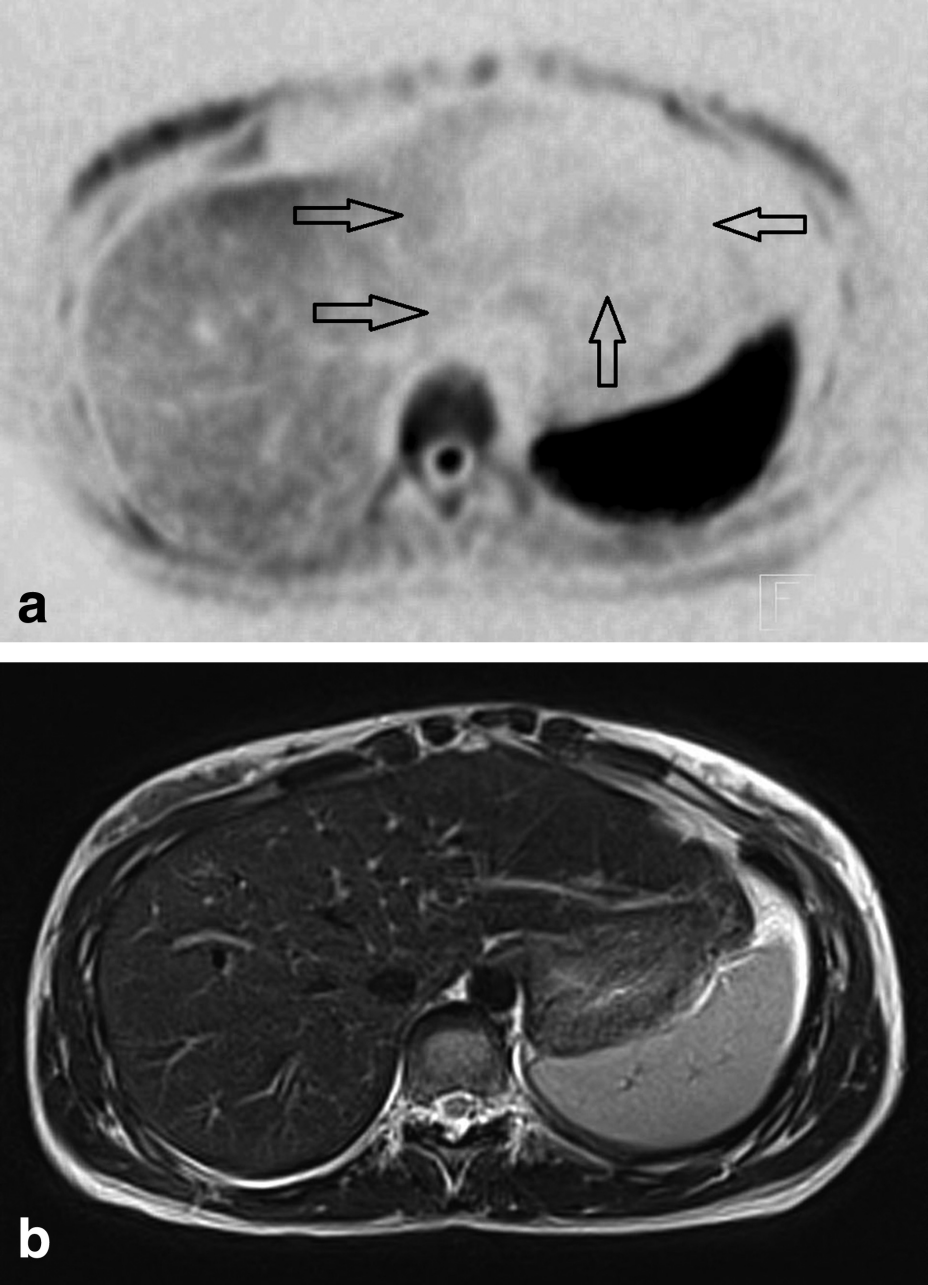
Axial b-500 image (a) demonstrates loss of signal within the left aspect of the liver from cardiac pulsation. The anatomy is not obscured on the axial T2 (b) image. This loss of signal is less problematic at low b-values and can be practically minimized by increasing the number of averages for DWI, or cardiac gating.
